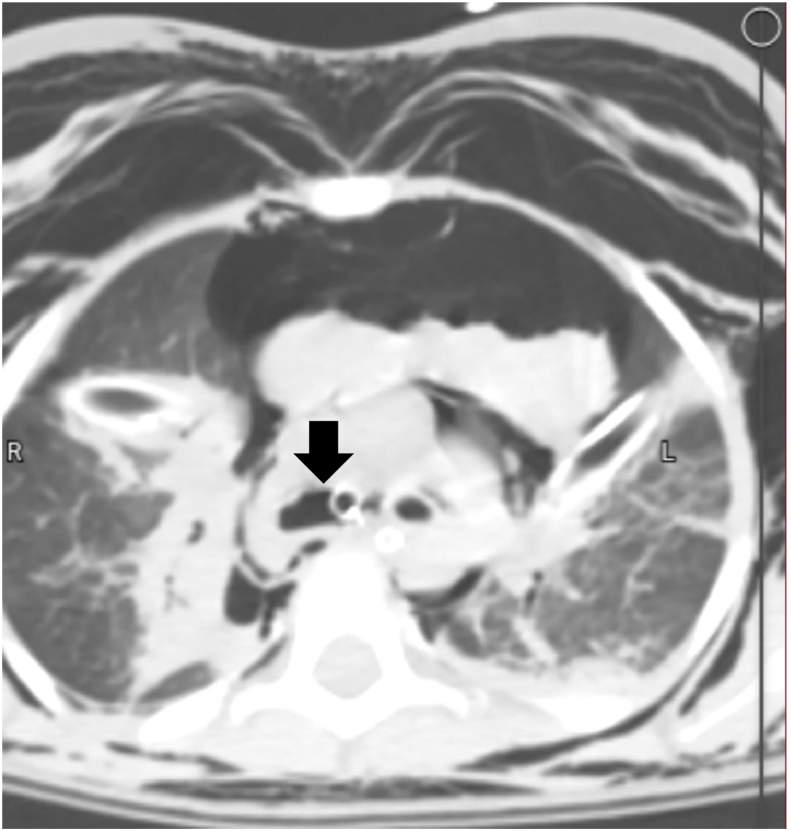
Axial view of thoracic CT scan (lung window) at the carina level, which demonstrates right main bronchus avulsion (arrow).
